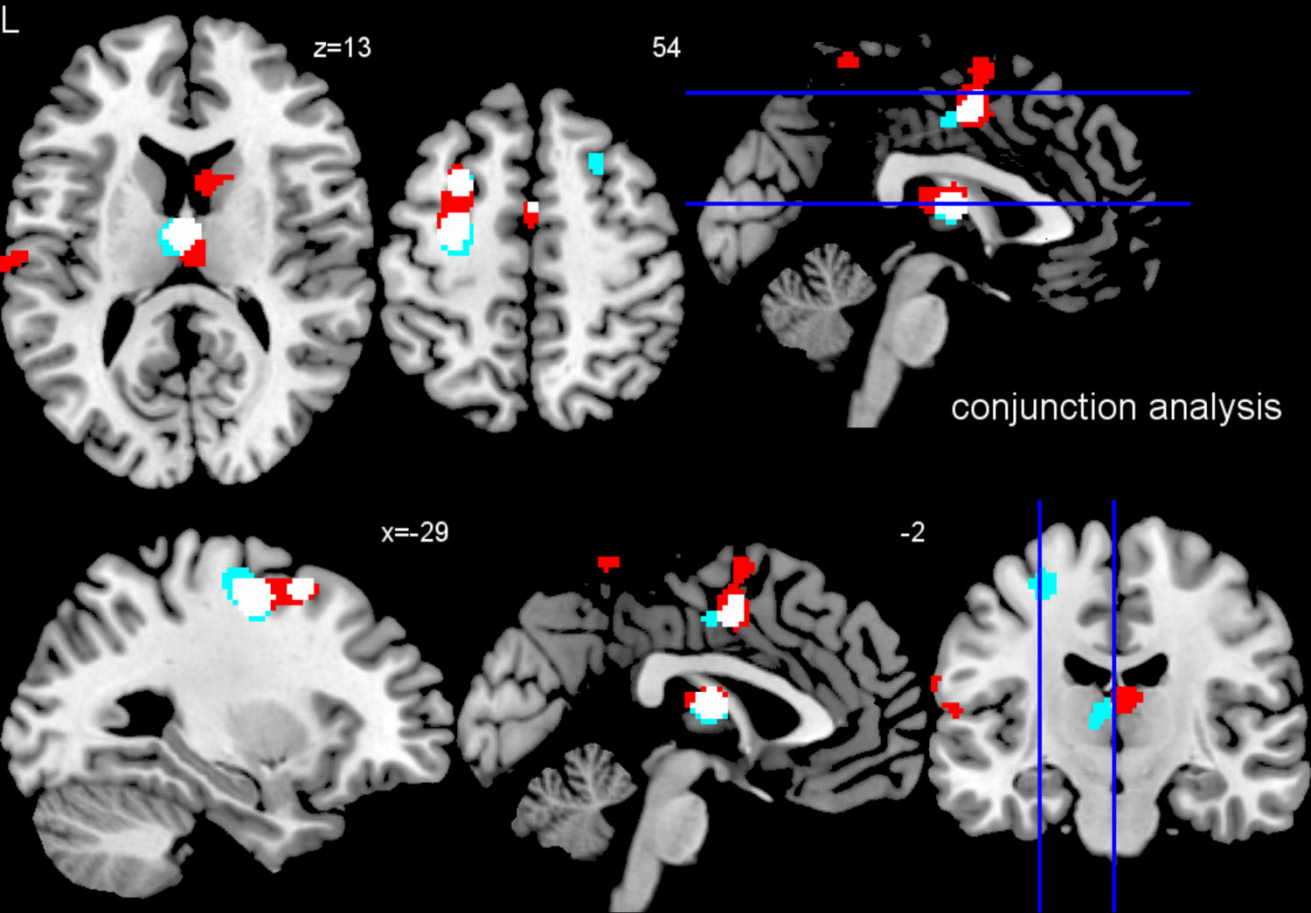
Conjunction analysis. Conjunction analysis for impaired brain regions in CBS and CBD in comparison with healthy control subjects–anatomical likelihood estimates meta-analyses. White clusters indicate overlap of both meta-analyses (CBS and CBD). Atrophy was measured by MRI. Coordinates are reported in MNI space. *L* left
